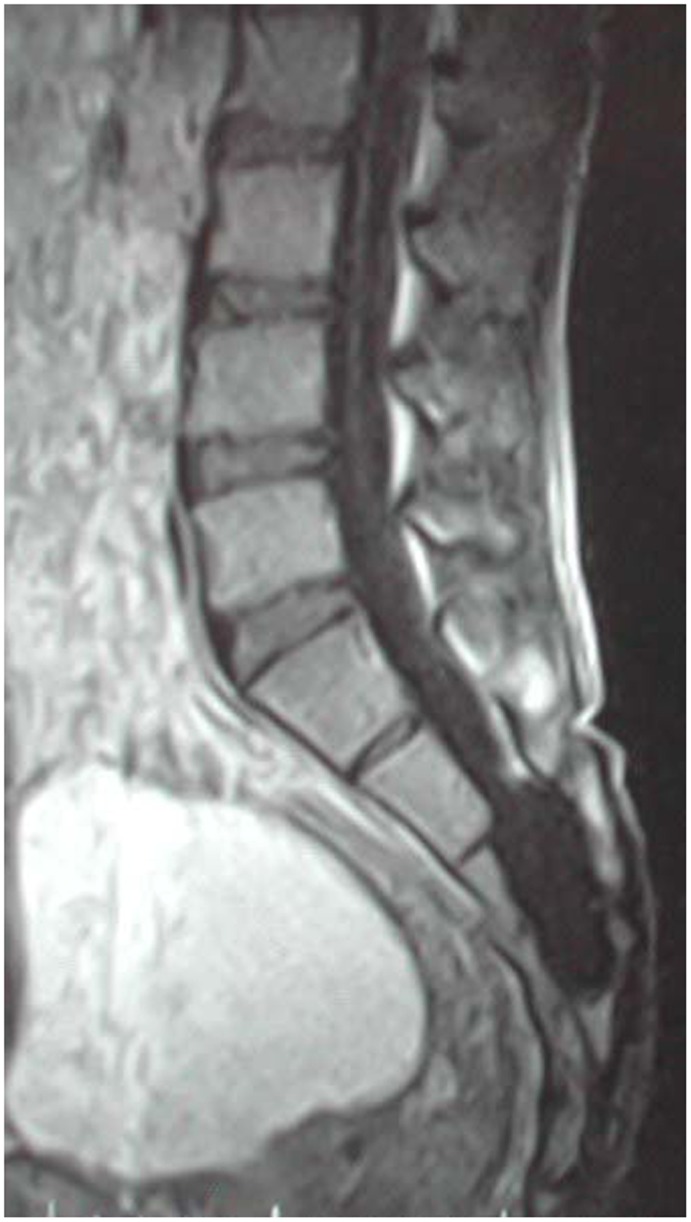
MRI findings of a cyst in the sacral spinal canal and low-lying tethered cord with syringomyelia.
